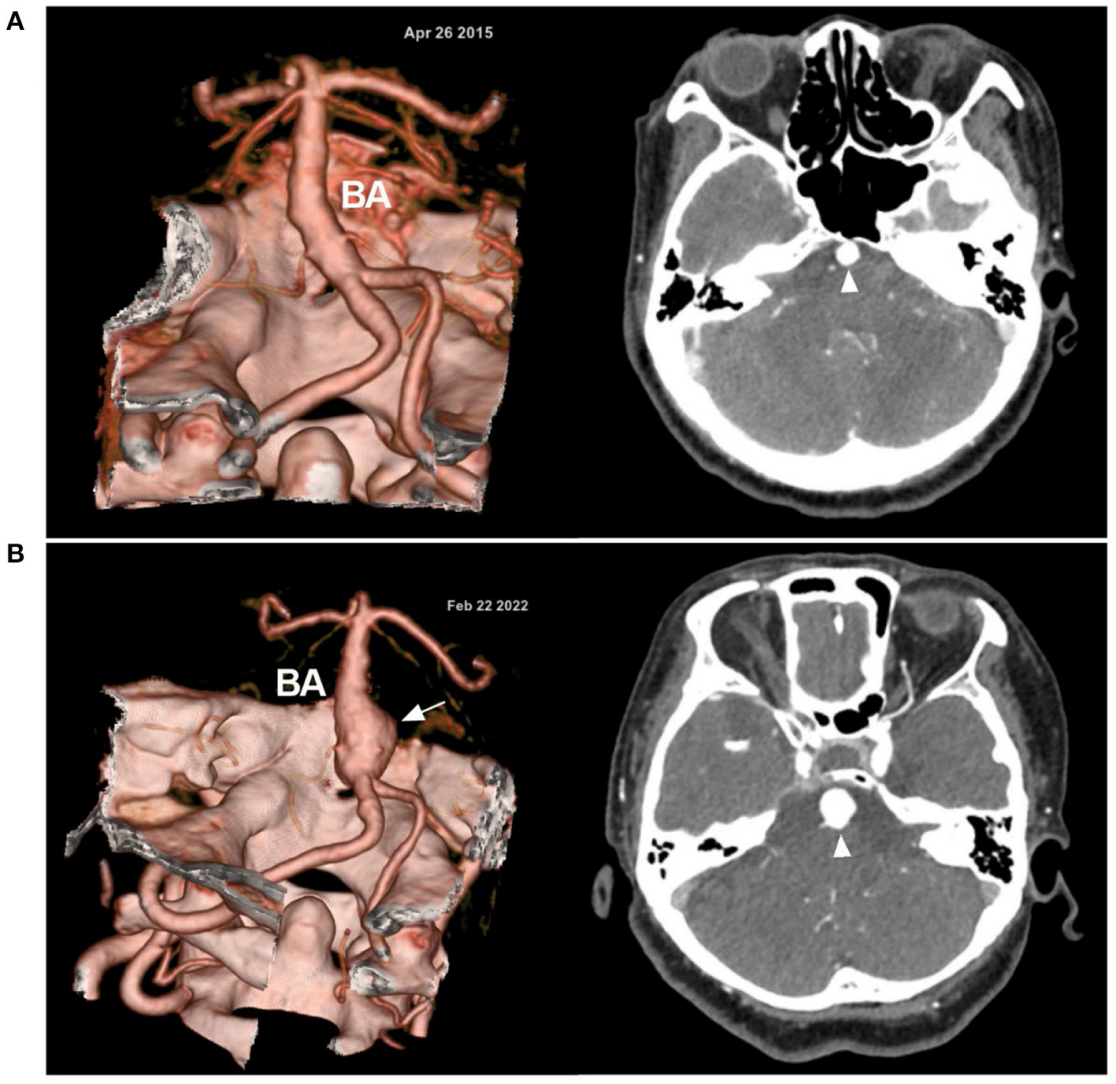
Progressive VBD in CTA. (A) In April 2015, CTA (left) showed the VBD; on enhanced CT (right), the BA (arrowhead) is highlighted. (B) In February 2022, CTA (left) after 7 years shows the progression of the VBD with the formation of a fusiform aneurysm (arrow); on enhanced CT (right), a larger BA (arrowhead) is highlighted. Over the 7 years, this female patient was always asymptomatic; now she is 61 years old. BA, basilar artery; CT, computed tomography; CTA, computed tomography angiography; VBD, vertebrobasilar dolichoectasia.
